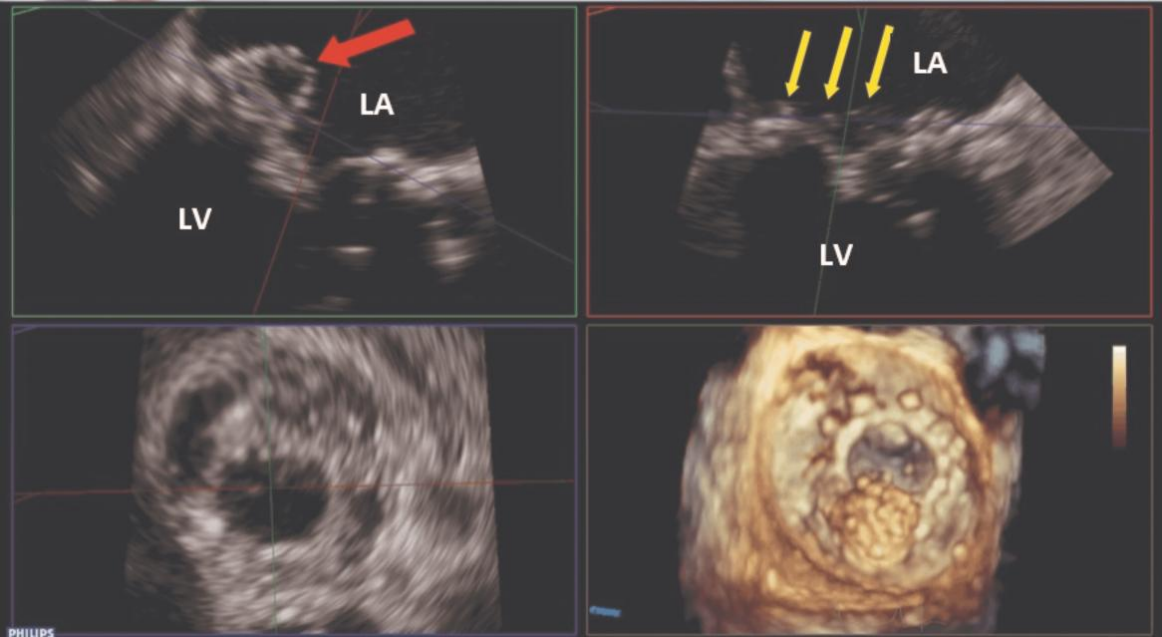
Multiplanar reconstruction of the bioprosthetic mitral valve using transoesophageal echocardiography demonstrates an abscess (red/single arrow) and multiple vegetations (yellow/three arrows). LA, left atrium; LV, left ventricle.
